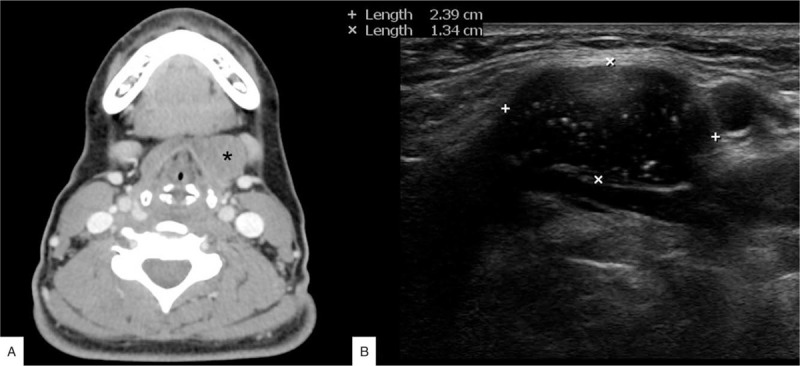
(A) Preoperative computed tomography (CT) scan. A 2.4 cm sized benign-appearing mass in the left infrahyoid region of the neck is noted, compressing the strap muscle (asterisk: mass). (B) Preoperative ultrasonographic findings. A thin-walled, heterogenous hypoechoic mass with internal hyperechoic debris is visualized.
